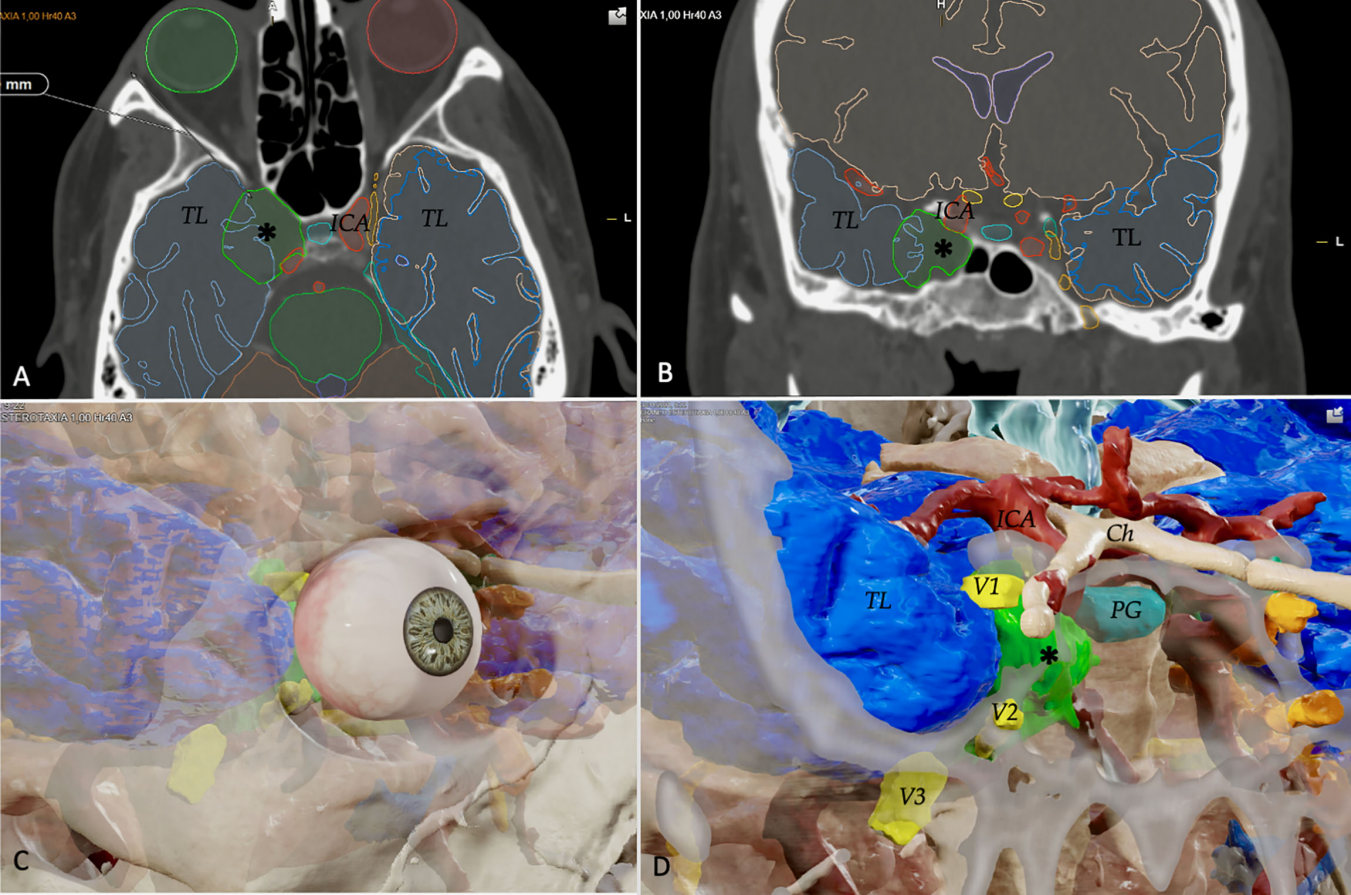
In Step 4, all preclinical data can be transferred to preoperative surgical planning to develop endoscopic transorbital approach. This is an example of a 3D model of a patient’s MRI with a cavernous sinus hemangioma, using the Brainlab (Brainlab Curve, Feldkirchen, Germany) navigation system to evaluate the presurgical planning. The system could highlight all the relevant neurovascular structures in the preoperative image in the axial (A) and coronal planes (B): for example, the temporal lobes (TL), the tumor (black asterisk), and the internal carotid artery (ICA). From the MRI, it is possible to create a 3D model of the patient, to determine, basing on our anatomical data and studies, the feasibility and potential efficacy of the endoscopic transorbital approach in this case (C, D). The location of the tumor (black asterisk) and other neurovascular, from a transorbital perspective, could be clearly seen: the three trigeminal branches (V1, V2, V3), the temporal lobe (TL), the internal carotid artery (ICA), the optic chiasm (Ch), and the pituitary gland (PG).
